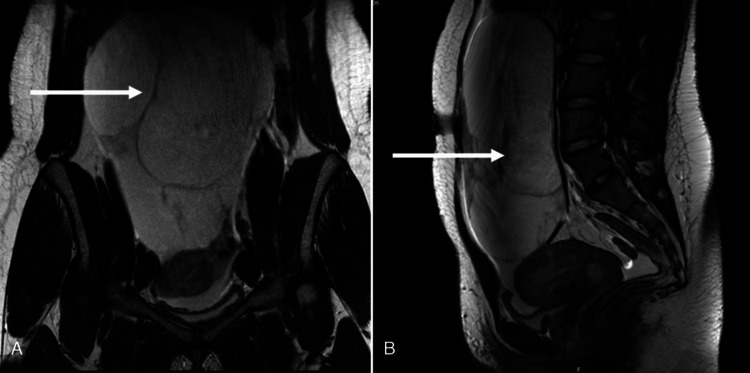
(A-B) Coronal and sagittal MRI images of the pelvic mass (arrows)
